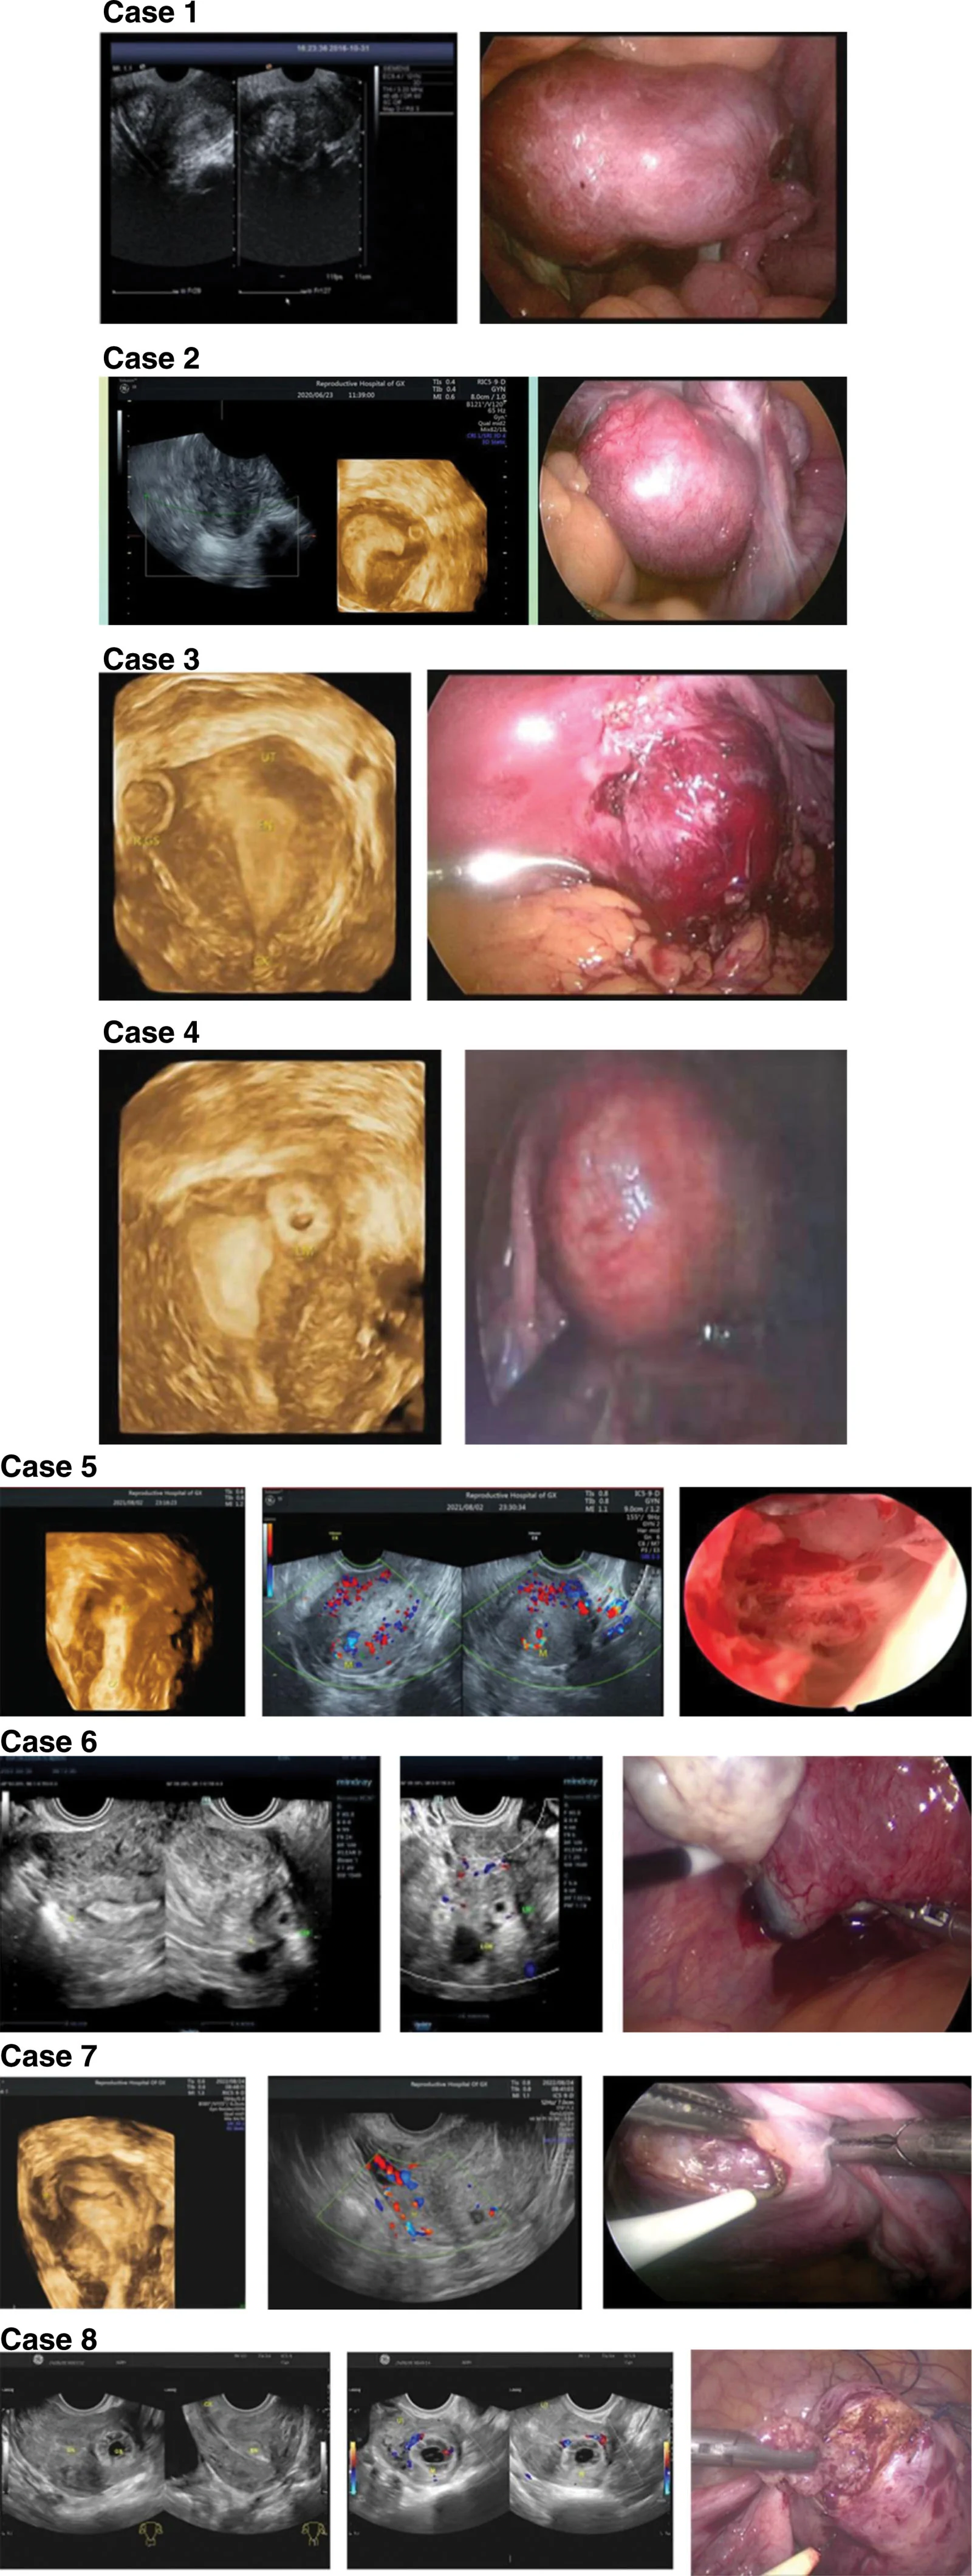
Preoperative ultrasound images and intraoperative surgical images (Cases 1–8).
